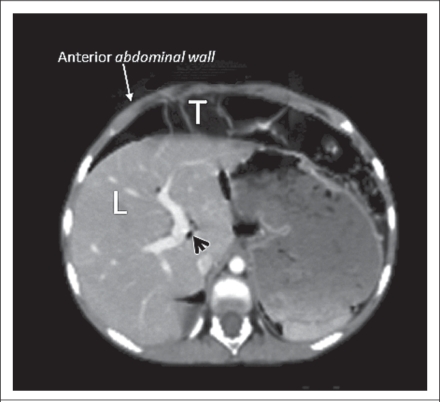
Coronal CT image showing hepatic portal venous gas (black arrow-head) and chilaiditi syndrome
